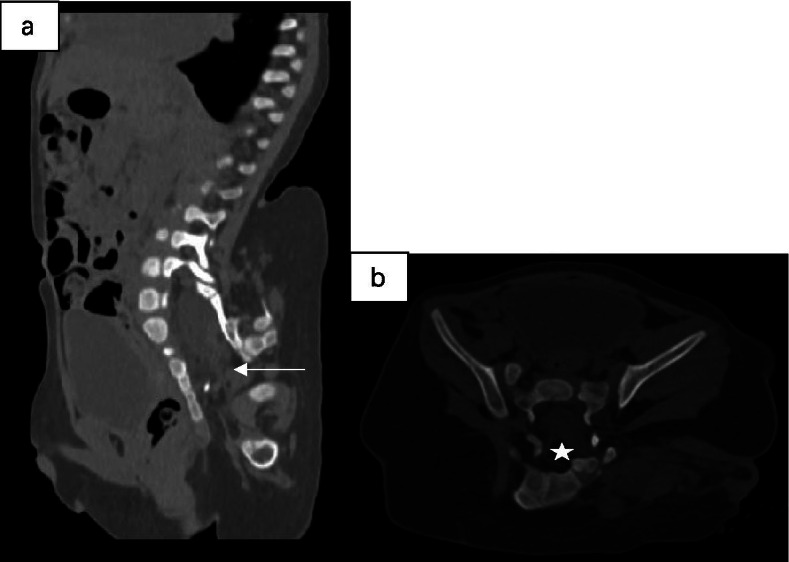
a and b sagittal and axial CT images showed Spinal dysraphisms of the sacrum (arrow and star)
